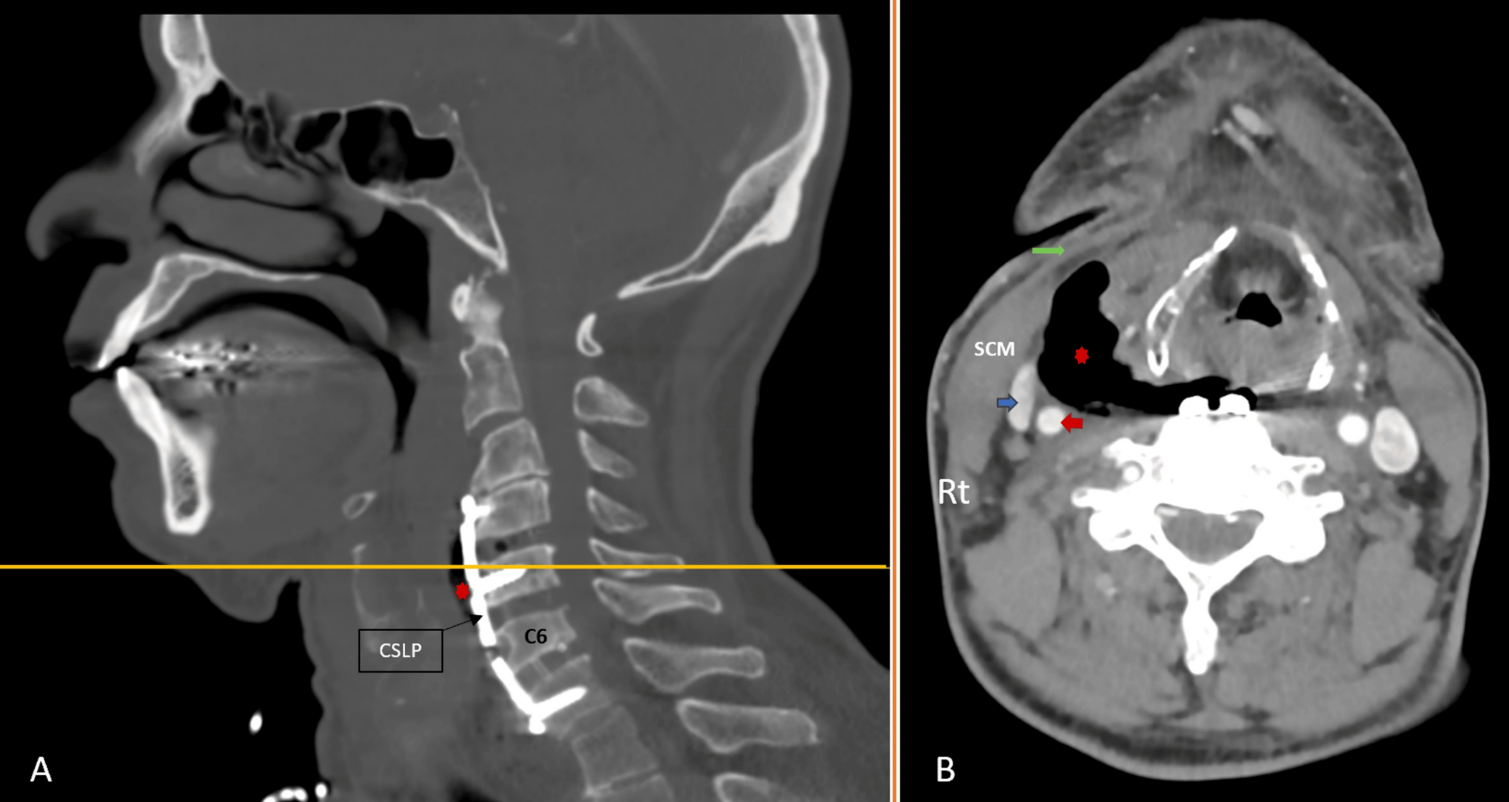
Neck CT with IV contrast taken upon the patient’s presentation with dysphagia and fever (A) Sagittal view showing the surgical fusion with a cervical spine locking plate (CSLP), and screws. Note the air at the prevertebral area (red star). (B) Axial view taken at the level of the fifth cervical vertebra (the yellow line in (A)). It shows the presence of an abscess evident by the presence of air (red star), and air-fluid level at the base. Notice that the air/abscess is present within the surgical plane: below the platysma (green arrow), and medial to the sternocleidomastoid muscle (SCM). The red and blue arrows are pointing at the common carotid artery, and the internal jugular vein, respectively.
